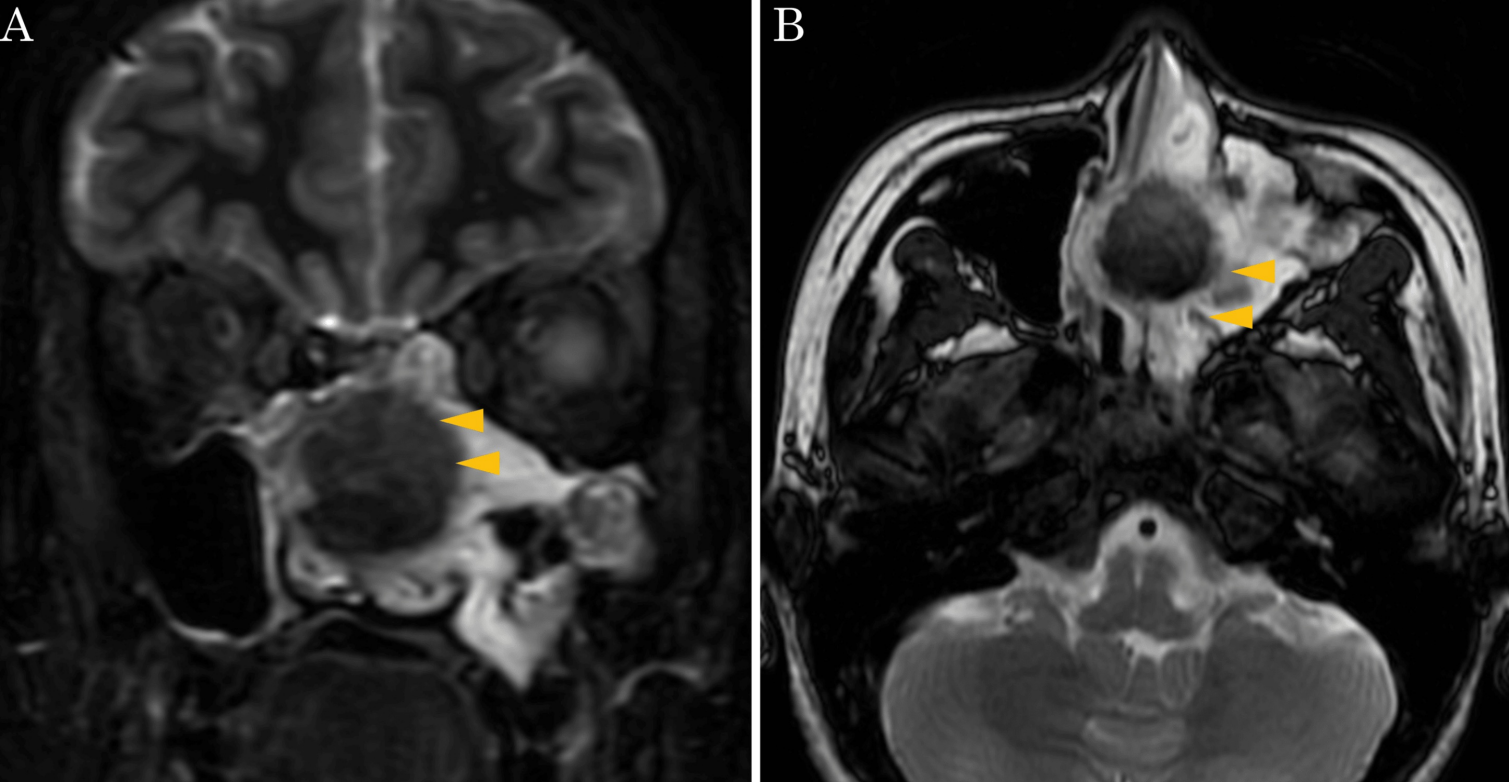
Sinus MRI Coronal (A) and axial (B) T2-weighted images show a mass lesion with low-intensity signals (arrowhead) in the left nasal cavity and maxillary sinus. MRI: magnetic resonance imaging
